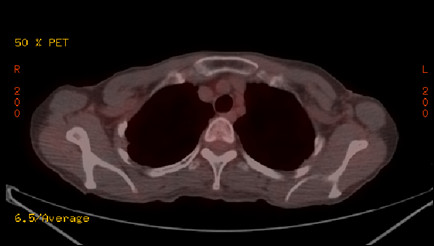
After administration of three cycles of combination chemotherapy using weekly paclitaxel and trastuzumab, the lymph node swelling disappeared and there was no apparent accumulation of FDG.
